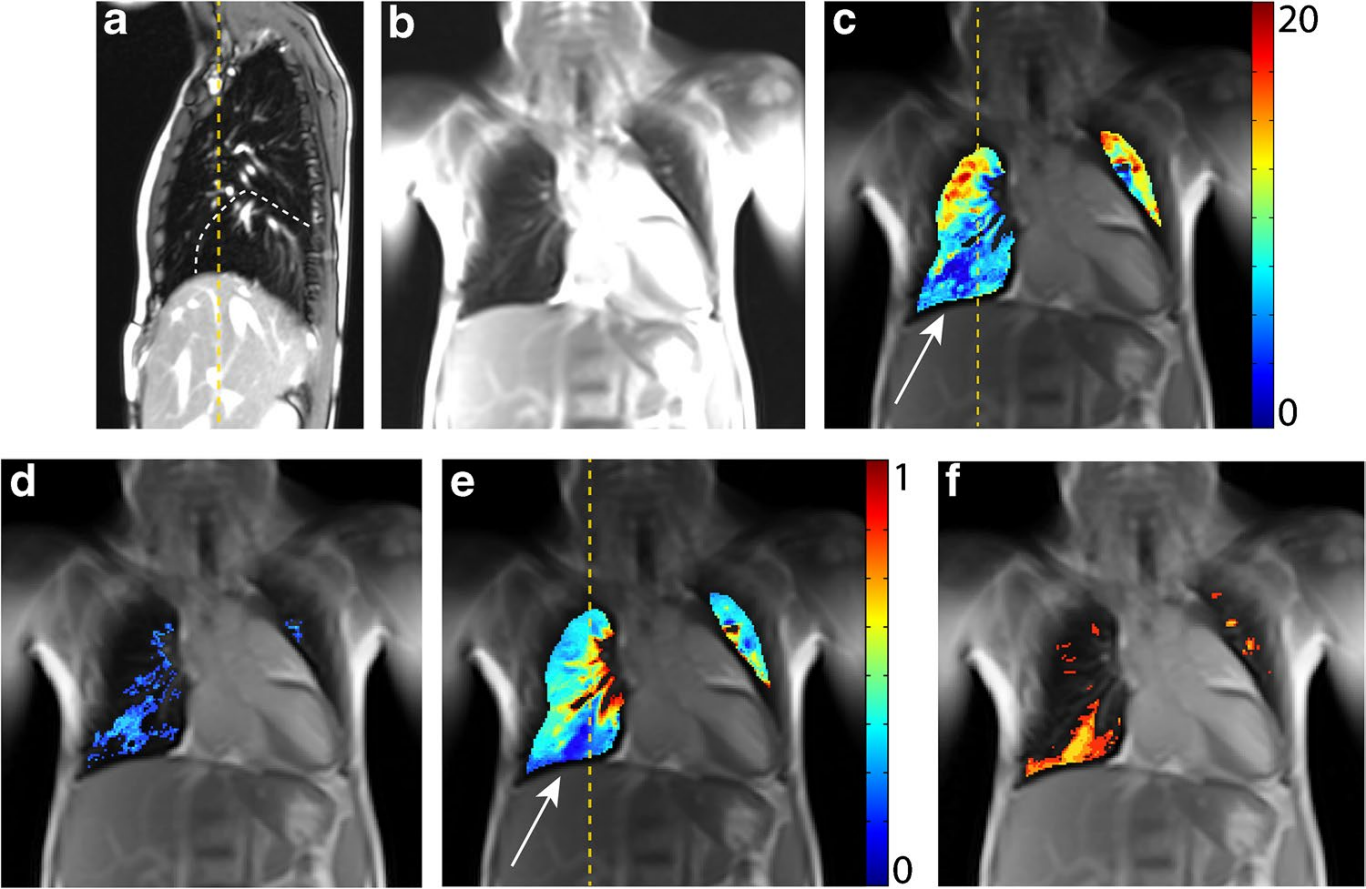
A 10-year-old girl following lobectomy of the right lower lobe for CPAM type 1 with an increased lung clearance index of 8.23 turnovers. A sagittal T2-weighted image (a) (repetition time [TR]/echo time [TE] 3.3/1.3 ms) and (b) coronal T1/2-weighted image (TR/TE 1.5/07 ms) demonstrate that the middle lobe replaces the area of the former right lower lobe. c-f Coronal functional magnetic resonance imaging maps: (c) fractional ventilation, (d) fractional ventilation defect percentage (brighter blue areas represent reduced function), (e) relative perfusion and (f) relative perfusion defect maps (brighter orange areas represent reduced function) show a local reduced fractional ventilation (c), while the overall fractional ventilation defect percentage is within the norm and an increased perfusion defect percentage (22.8%) predominantly in the area of the right middle lobe (e) (*white arrow*). *Yellow dashed lines* indicate plane levels. *CPAM* congenital pulmonary airway malformation
